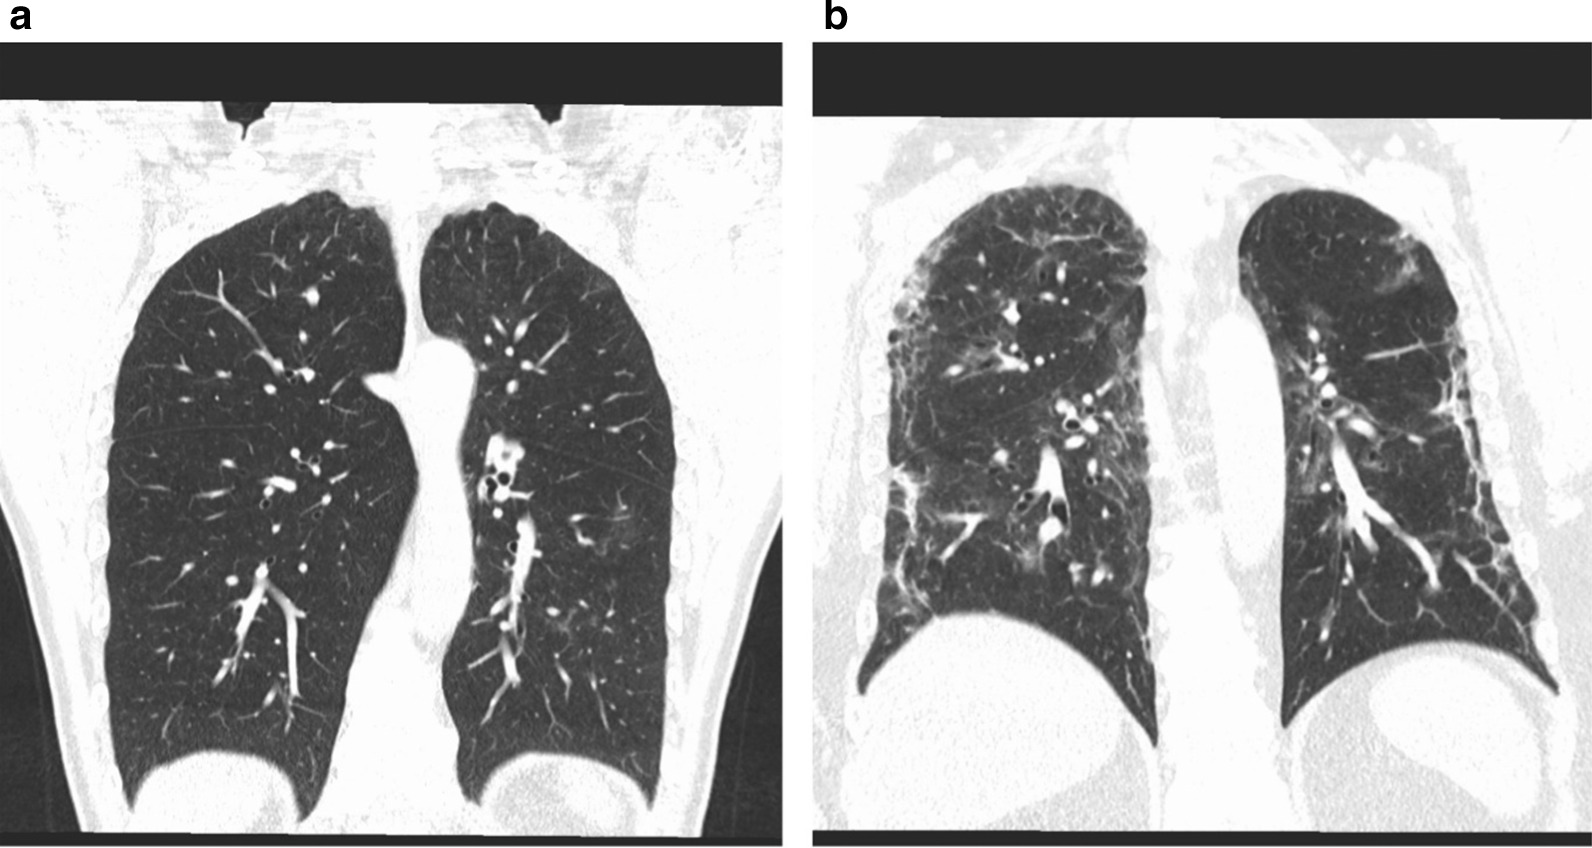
Representative CT scans of COVID-19 patients with or without hyperferritinemia. When comparing lung pathologies in CT scans 60 days after COVID-19 onset, patients with persisting hyperferritinemia presented with significantly more severe lung pathologies. A representative CT scan of two individuals without (a) and with (b) hyperferritinemia are shown
